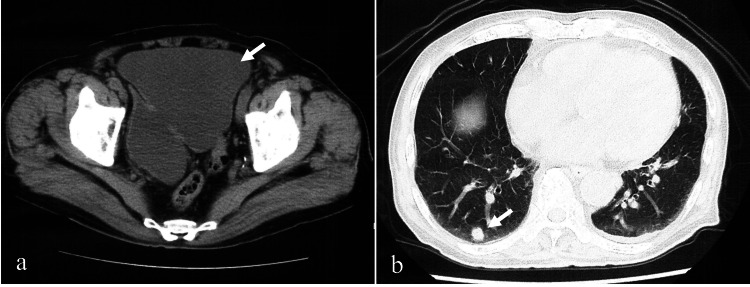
Pelvic and chest computed tomography of case 2. Abdominal computed tomography revealed chronic bladder retention and urinary retention throughout the disease course (a, arrow), along with the presence of a bladder diverticulum. Chest CT taken at 18 months into the disease course shows a solitary lung metastasis without associated symptoms (b, arrow).
